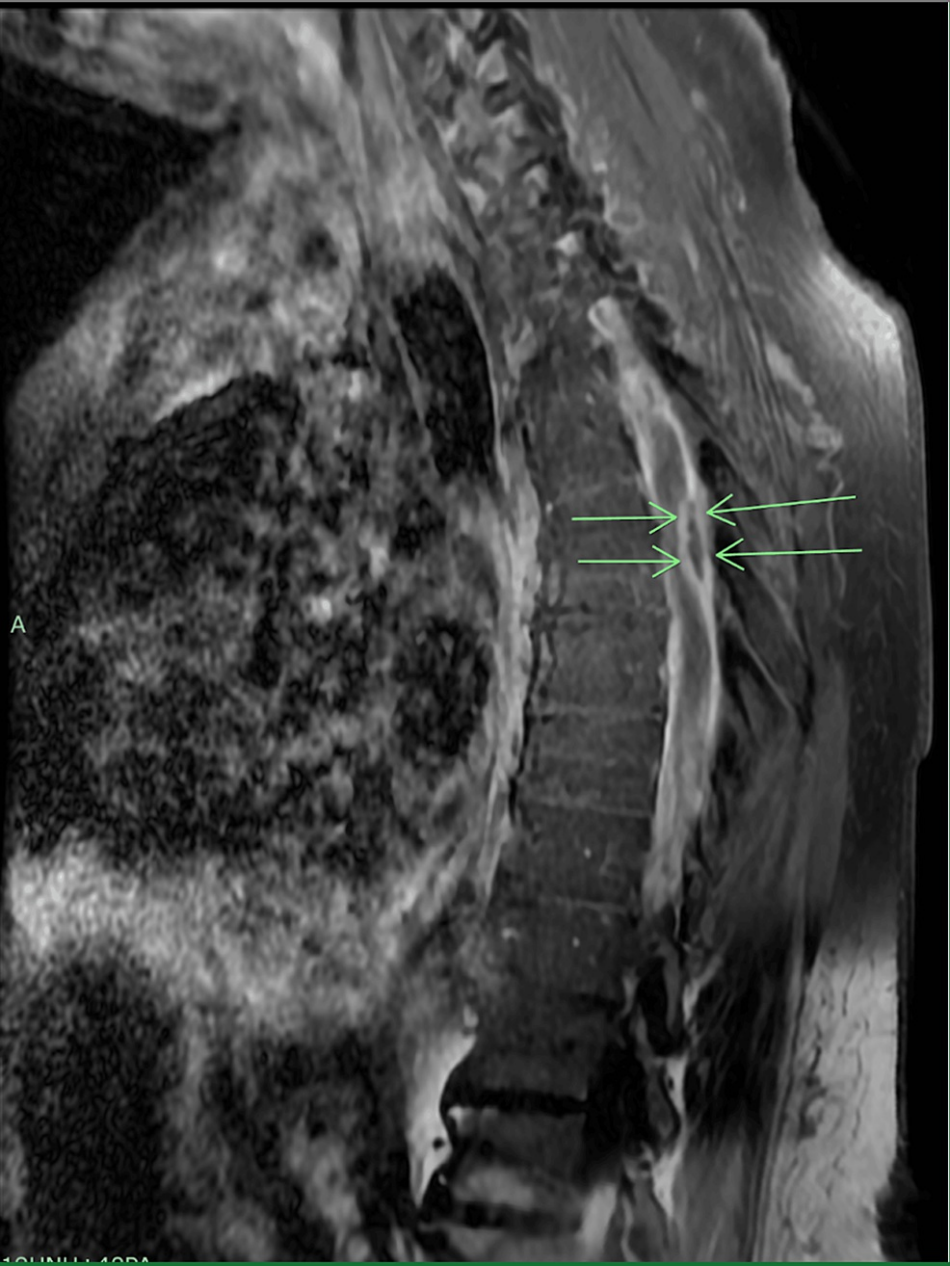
Sagittal post-contrast T1 MRI with phlegmon and spinal cord edema At the T6 level, the T1 sagittal post-contrast with fat saturation demonstrated diffuse subdural enhancement throughout the thoracic spinal canal, consistent with phlegmon. In addition, there is a 2.4x0.7 cm posterior subdural rim enhancing fluid collection at the T6 level that is consistent with intradural abscess. The abscess exerts a mass effect on the spinal cord and was clinically correlated with deficits in the patient's neurological exam.
